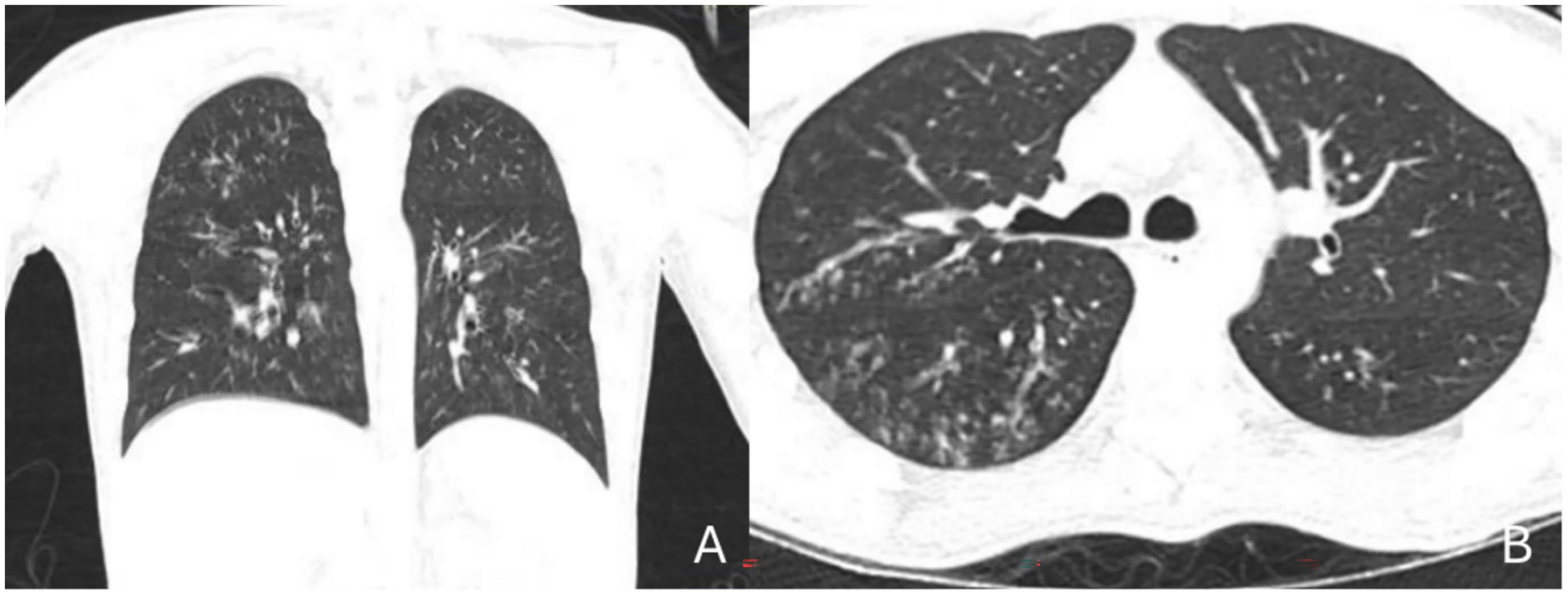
Chest computed tomography (CT). (A) Coronal view; (B) Axial view: (A) Symmetrical bilateral involvement is confirmed, showing a ground-glass opacity pattern with interstitial thickening, predominantly affecting the middle and lower lobes. No pleural effusions or visible mediastinal masses are observed. This coronal view highlights the classic “inverted butterfly wings” distribution pattern, characteristic of immunosuppressed patients. (B) Bilateral ground-glass opacities are noted, suggestive of atypical interstitial pneumonia, highly compatible with infective process in an immunocompromised patient. The pattern is centrally and peribronchially predominant, accompanied by bronchial wall thickening and a fine reticulonodular appearance. No lobar consolidations, cavitations, or mediastinal lymphadenopathy are identified.
